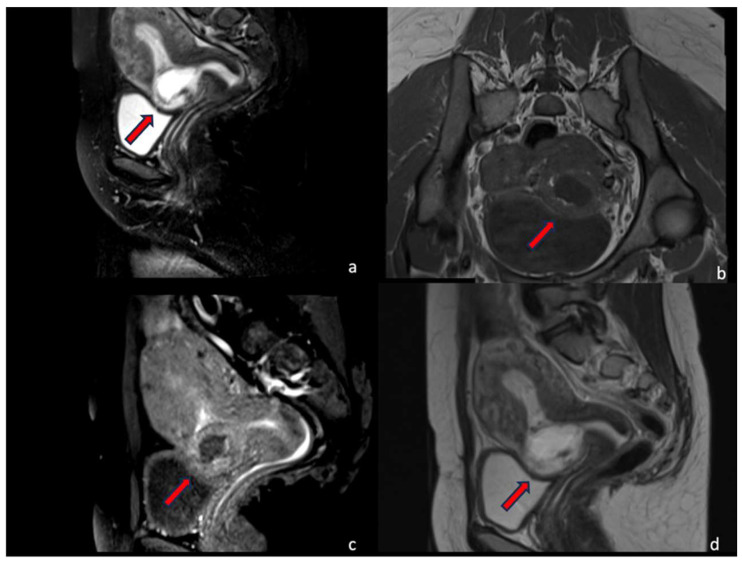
CSP Type II. Sagittal T2-weighted fat-saturation, (a) axial T1-weighted, (b) sagittal T1-weighted fat-saturation, and (c) sagittal T2-weighted (d) MRI sequences of the pelvis showed a gravid uterus with placental implant at the isthmus (red arrows) in the previous cesarean section scar. Placenta invaded the entire thickness of the myometrium and reached the serosa without any signs of interruption. A clear cleavage point with the posterior wall of the bladder was not visible.
